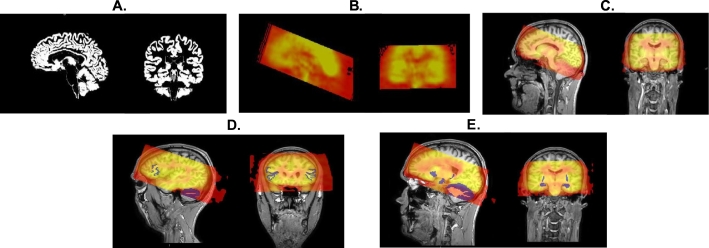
Panel A. shows gray matter segmentation image derived from the T1 structural MRI image used for co-registration of the ^123^I-IDEX SPECT scan. Panel B. ^123^I-IDEX SPECT scan of the same subject showing high cortical binding with no binding in white matter and cerebrospinal fluid. Panel C. ^123^I-IDEX SPECT scan co-registered to subject's own T1 structural MRI image; darker areas are over white matter tracts, ventricles, and cerebellum showing no ^123^I-IDEX binding. Panel D. In blue masks created from a segmented FreeSurfer image of the dorsolateral prefrontal cortex (DLPFC) (region of interest B_s_), used for the assessment of M_1_ binding, and cerebellar gray matter (B_n_), overlaid over the SPECT scan in pseudo colors. Panel E. In blue, masks used for the assessment of M_1_ binding (B_s_) in the hippocampus, caudate nucleus and putamen.
